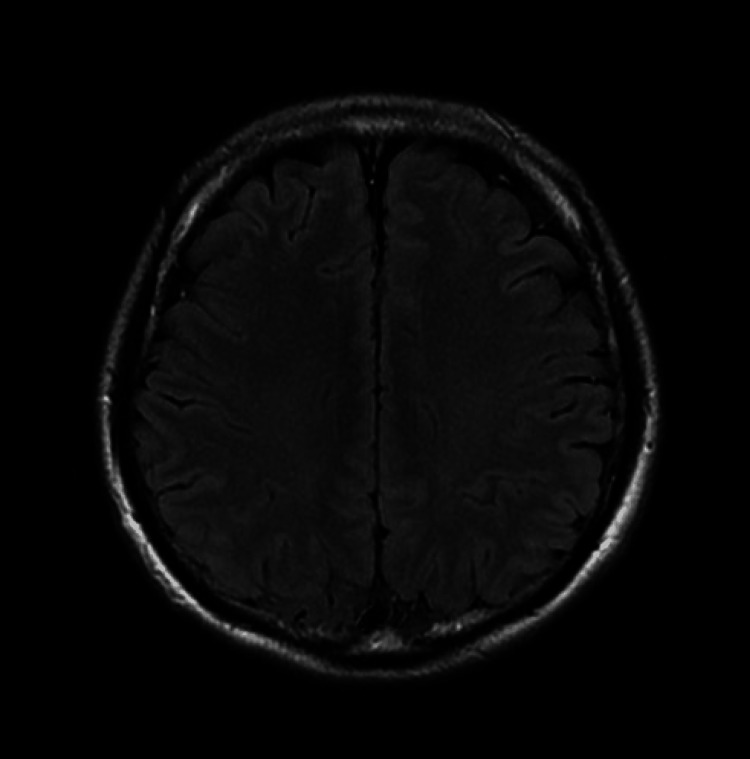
Axial FLAIR MRI obtained on post-stimulation day 17 The image demonstrates no structural abnormalities in the cerebral cortex or subcortical white matter FLAIR: fluid-attenuated inversion recovery; MRI: magnetic resonance imaging
